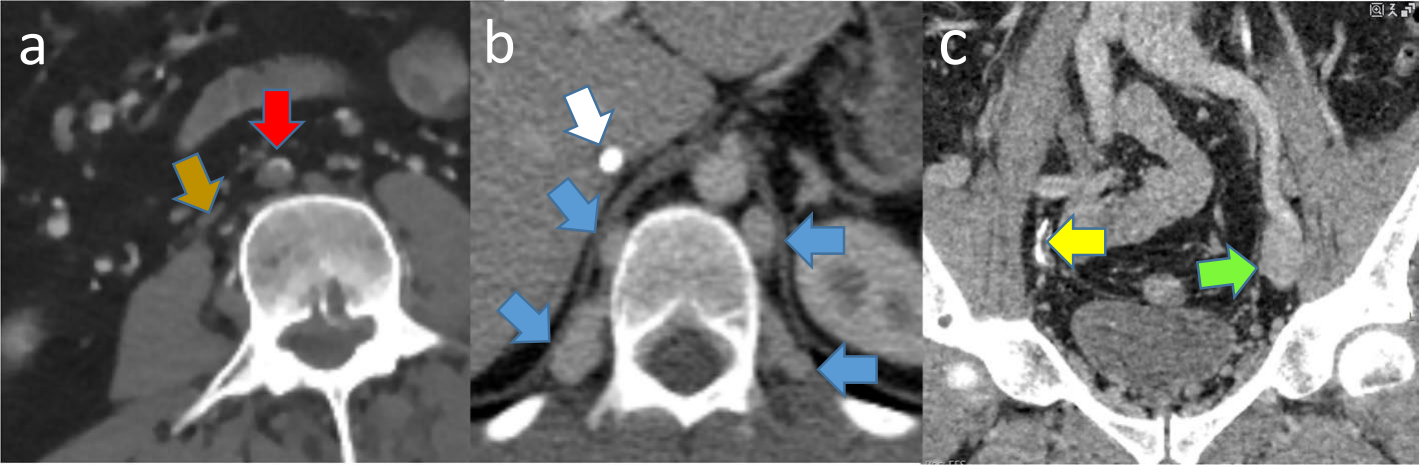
shows contrast enhanced CT scans with coloured arrows indicating important structures. a Proband 1 (older brother): Gracile VCI (brown), infrarenal aortic occlusion (red). b Proband 2 (younger brother): Calcified reminicents of VCI (white), dilated paravertebral collateral veins (blue). c Proband 3 (father): Normal left iliac vein (green). Agenetic/occluded(calcified right iliac vein (yellow)
